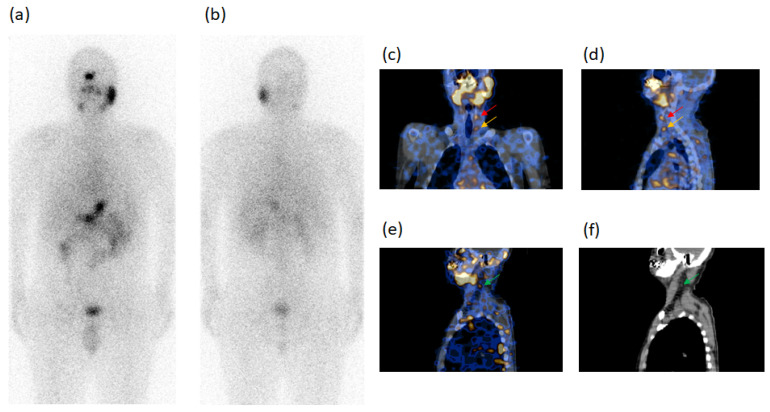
The same patient described in Figure 2 (case n. 2, Table 3) was re-evaluated in the follow-up. WBS in both anterior (a) and posterior (b) views did not show any radioiodine avid foci to consider as residues or malignant lesions. Fused SPECT/CT images in coronal (c) and left (d) and right sagittal (e) views excluded the two malignant foci evidenced in basal condition. However, it identified three new foci, two of which in the left laterocervical region (red and yellow arrows) and another in right laterocervical region, the latter better evidenced in sagittal right view (green arrow) and confirmed by CT (f). These foci were classified as lymph node metastases in disease progression. The patient was treated again with a therapeutic dose of radioiodine. Thyroglobulin levels in hypothyroidism condition: 36 ng/mL. AbTg: absent.
